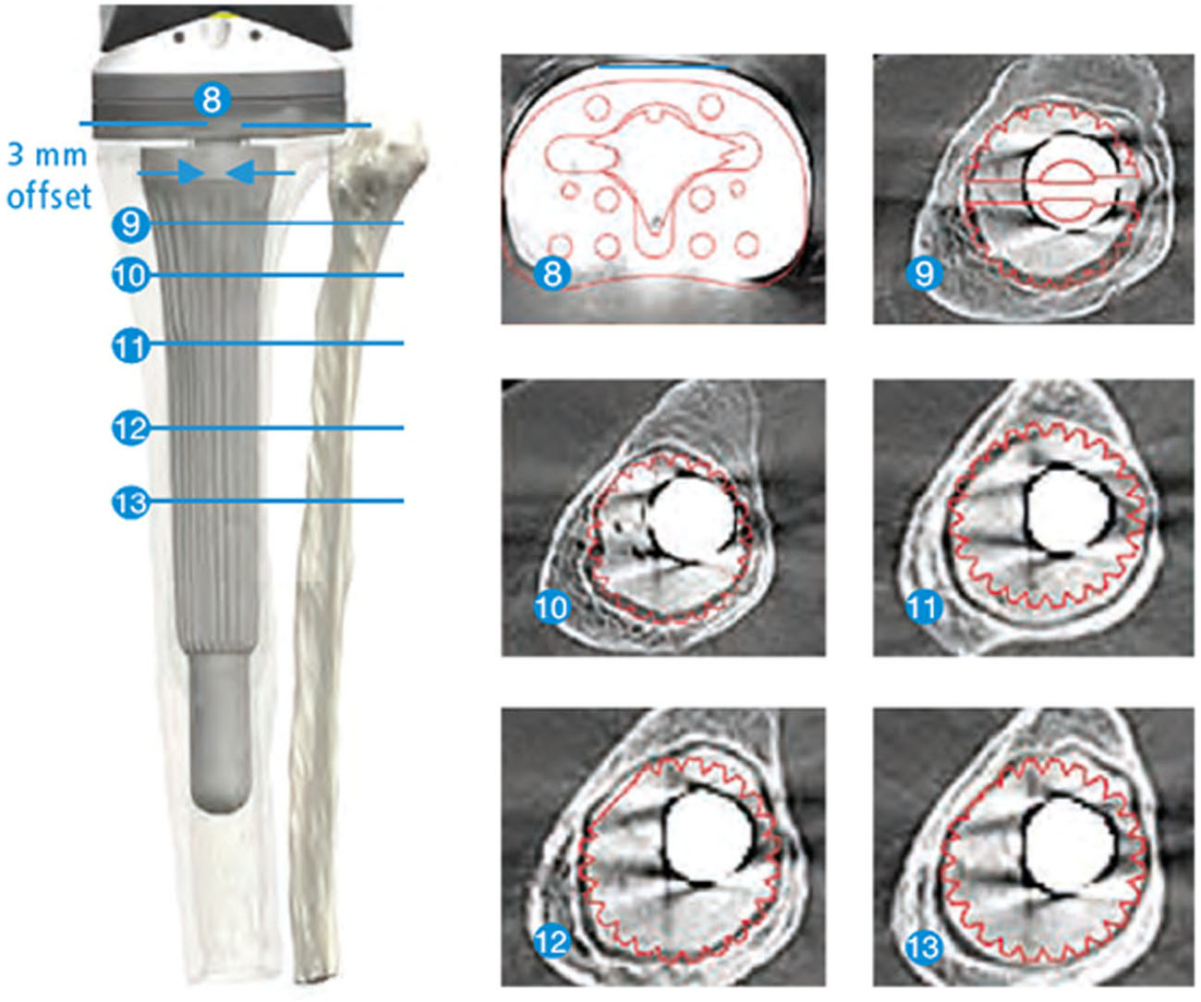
Radiological planning of the tibial implant based on CT scans including customised offset and stem alignment. The subfigures (8–13) on the right side demonstrate the axial view of the tibia from the corresponding markings on the left side. CT, computer tomography.
